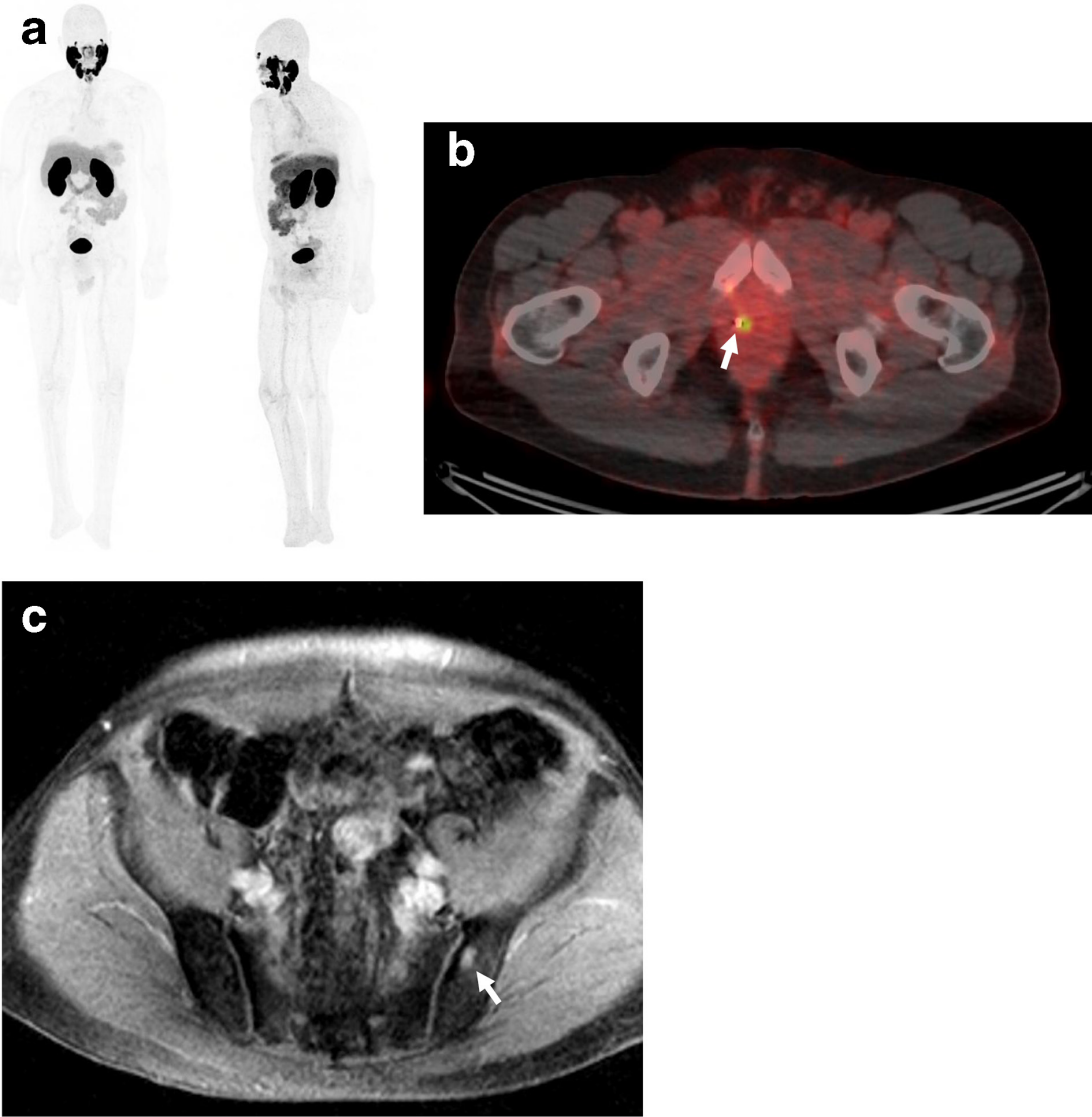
Whole body Prostate Specific Membrane Antigen Positron Emission Tomography (PSMA PET/CT, A), and axial slices of PSMA PET/CT (B) shows focal tracer uptake within the midline to right apical peripheral zone, without uptake in any previously defined prostate lesions, lymph nodes, or bone. MRI of the pelvis/prostate showed indeterminate area in the left ilium (C), without a correlate found on PSMA PET/CT or NaF PET/CT (not pictured).
